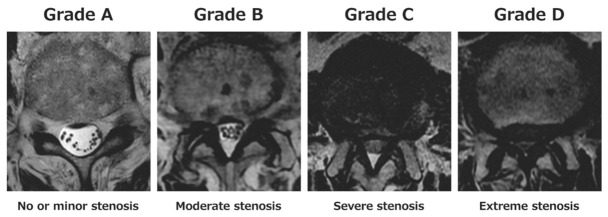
Schizas classification of lumbar spinal canal stenosis. Representative axial T2-weighted MRI showing Schizas Grades A–D. Grade A: clearly visible cerebrospinal fluid with dorsally arranged nerve rootlets; Grade B: rootlets occupying the dural sac, but individually identifiable; Grade C: a homogeneous gray signal without recognizable rootlets, but with preserved posterior epidural fat; and Grade D: complete obliteration of both rootlets and posterior epidural fat.
